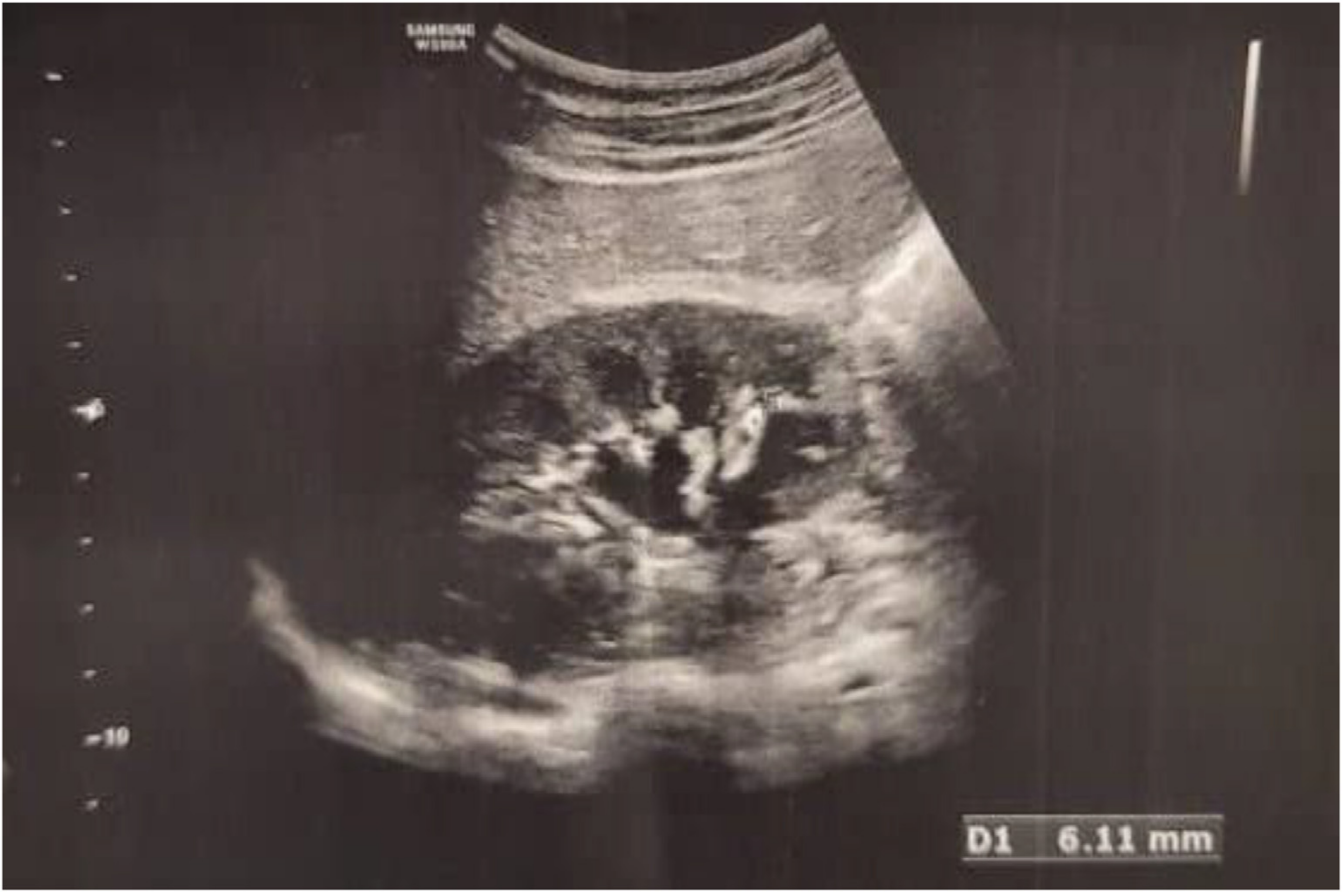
Kidney ultrasonography: Mild dilatation of pyelocaliceal system.
